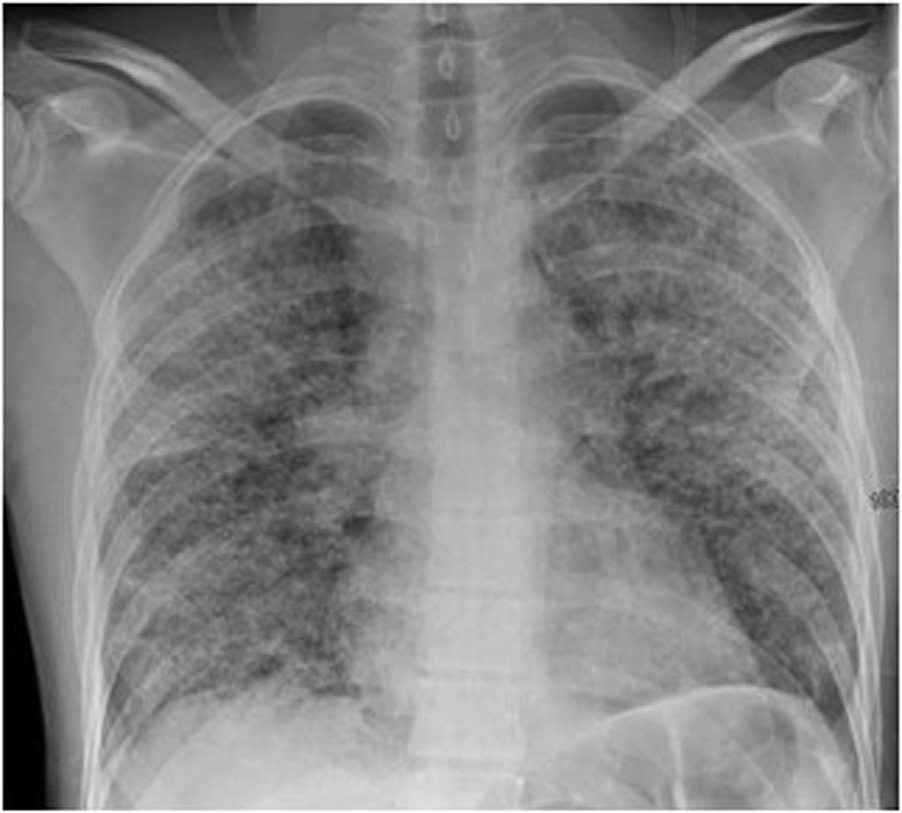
Chest radiograph on presentation to hospital without respiratory symptoms*.*
